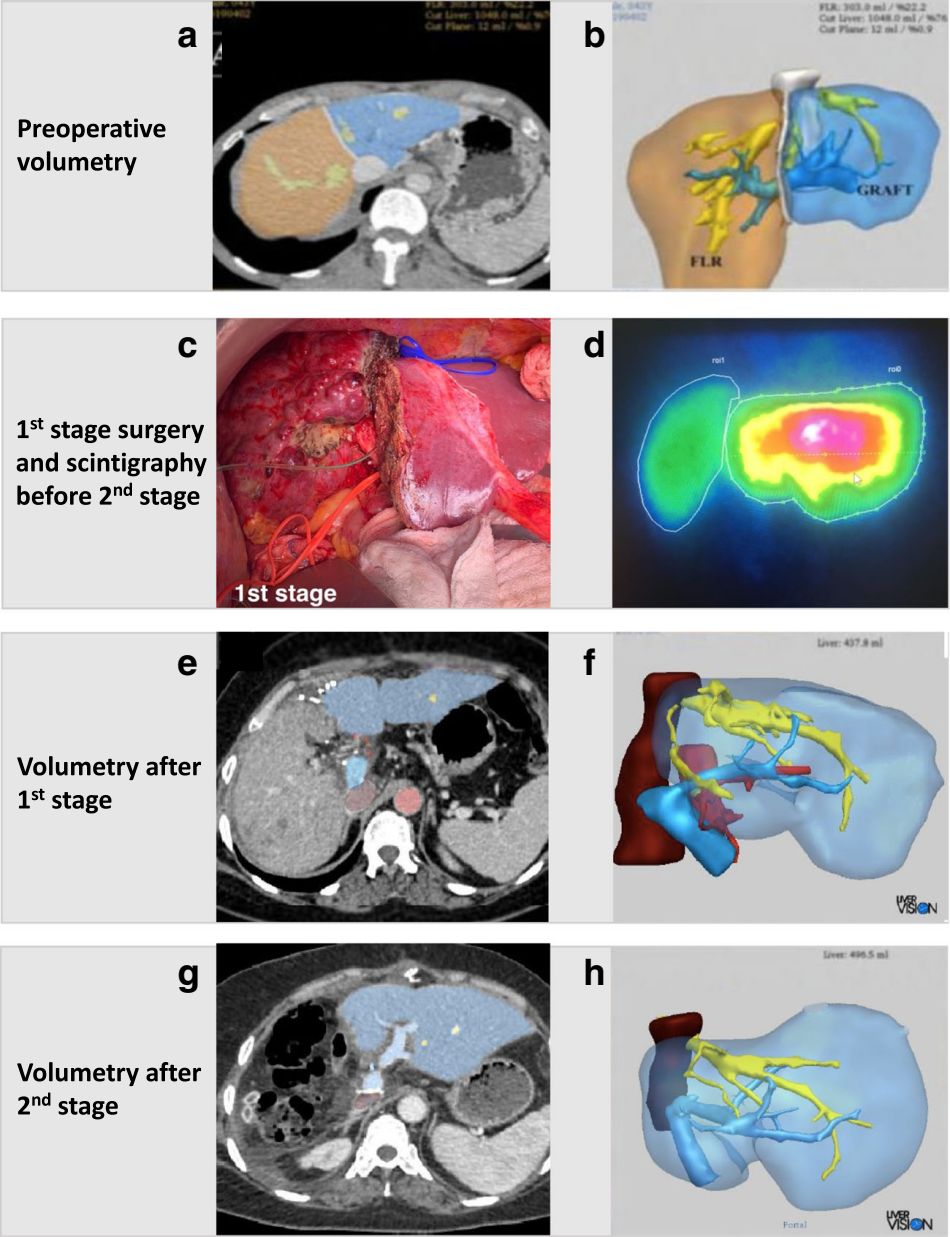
Clinical case of RAPID approach in a cirrhotic patient with portal hypertension and hepatocellular carcinoma (Ankara University School of Medicine) [106]. After removing segments 1–3 (A/B), a small left lobe graft (segments 2, 3, 4; graft-to-recipient weight ratio 0.35) was transplanted (C). Due to the portal hypertension, inflow modification was necessary and a hemiportocaval shunt was created that re-routed approximately two-thirds of portal flow to the inferior vena cava decreasing the portal pressure from 24 to 14 mmHg. After 22 days, hepatobiliary scintigraphy showed an almost 70% functional shift to the left lobe graft (D), and there was a 56% graft volume hypertrophy (E, F). In the second stage, the right hemi-liver was removed, with closure of the hemiportocaval shunt. To control potential portal hyperflow and arterial buffer response, splenic artery ligation was added at the end of the operation. The patient recovered without liver failure and is without tumor recurrence almost 2 years after the 1st-stage operation (G/H)
